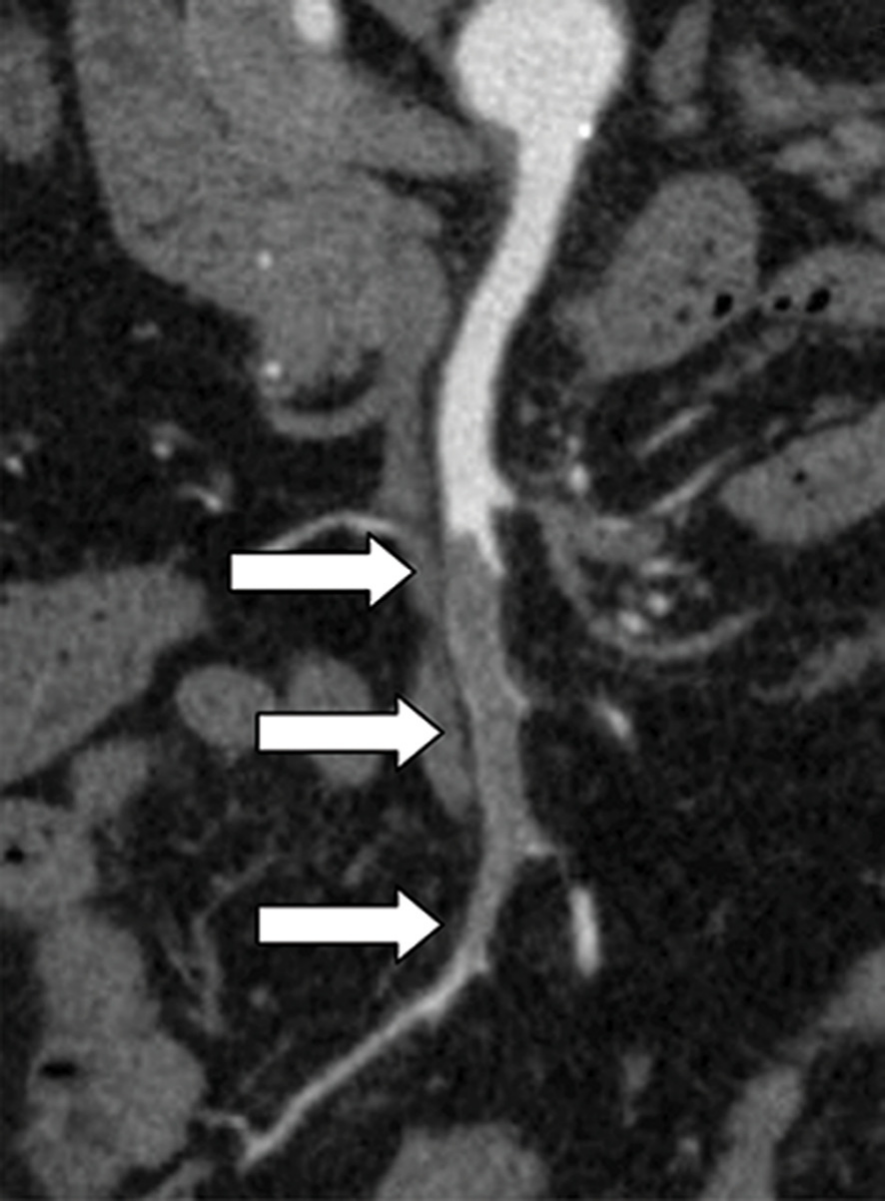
Curved multiplanar reconstruction of CT angiography showing a segmental occlusive acute embolism of the mid portion of the main trunk of superior mesenteric artery proximal to the ileocolic artery (arrows).
